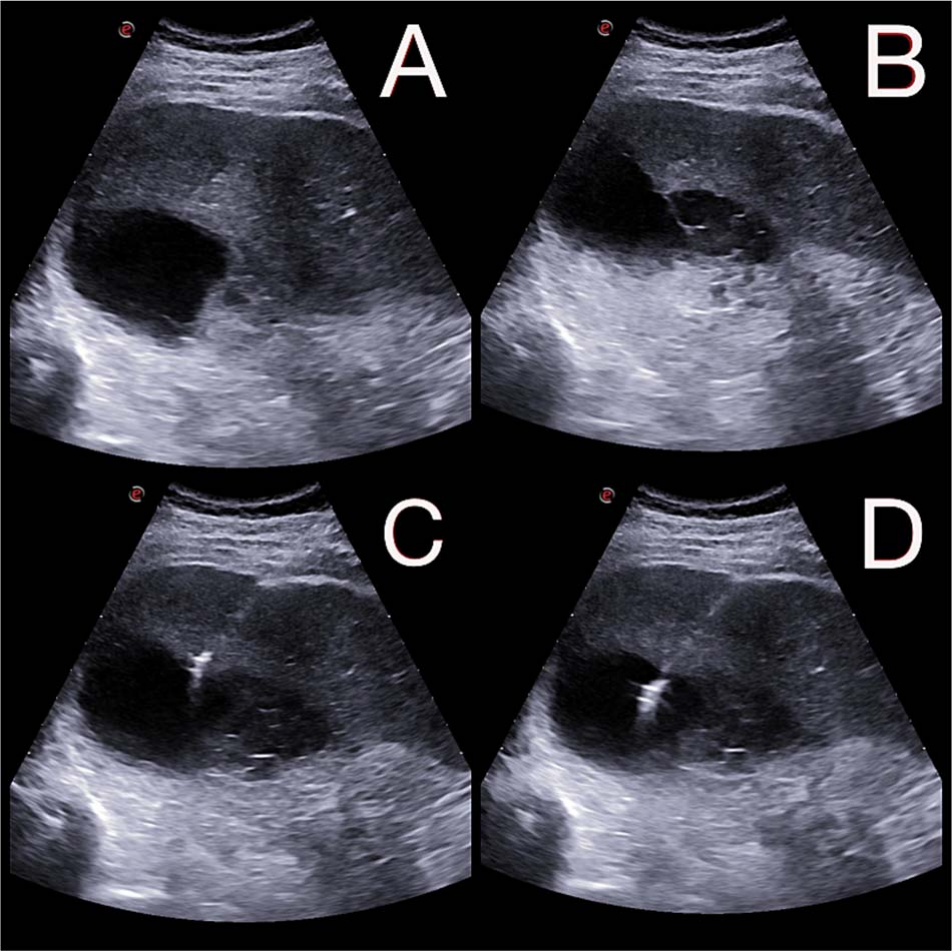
(A and B) Abdominal ultrasound depicting a 9 × 5 cm abscess in segment VII. (C and D) Percutaneous drainage performed with placement of a 10 Fr catheter under ultrasound guidance.
